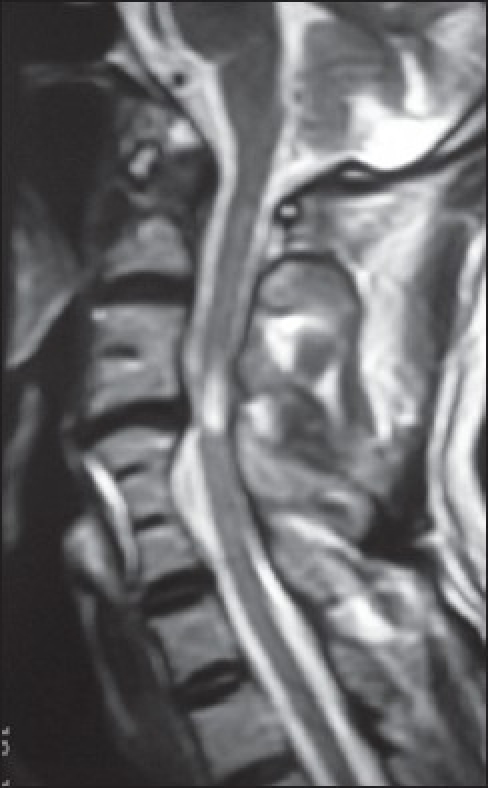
T2-weighted magnetic resonance image showing a large C4-5 disc prolapse resulting in compression of the dural tube and hyperintensity in the adjoining spinal cord
